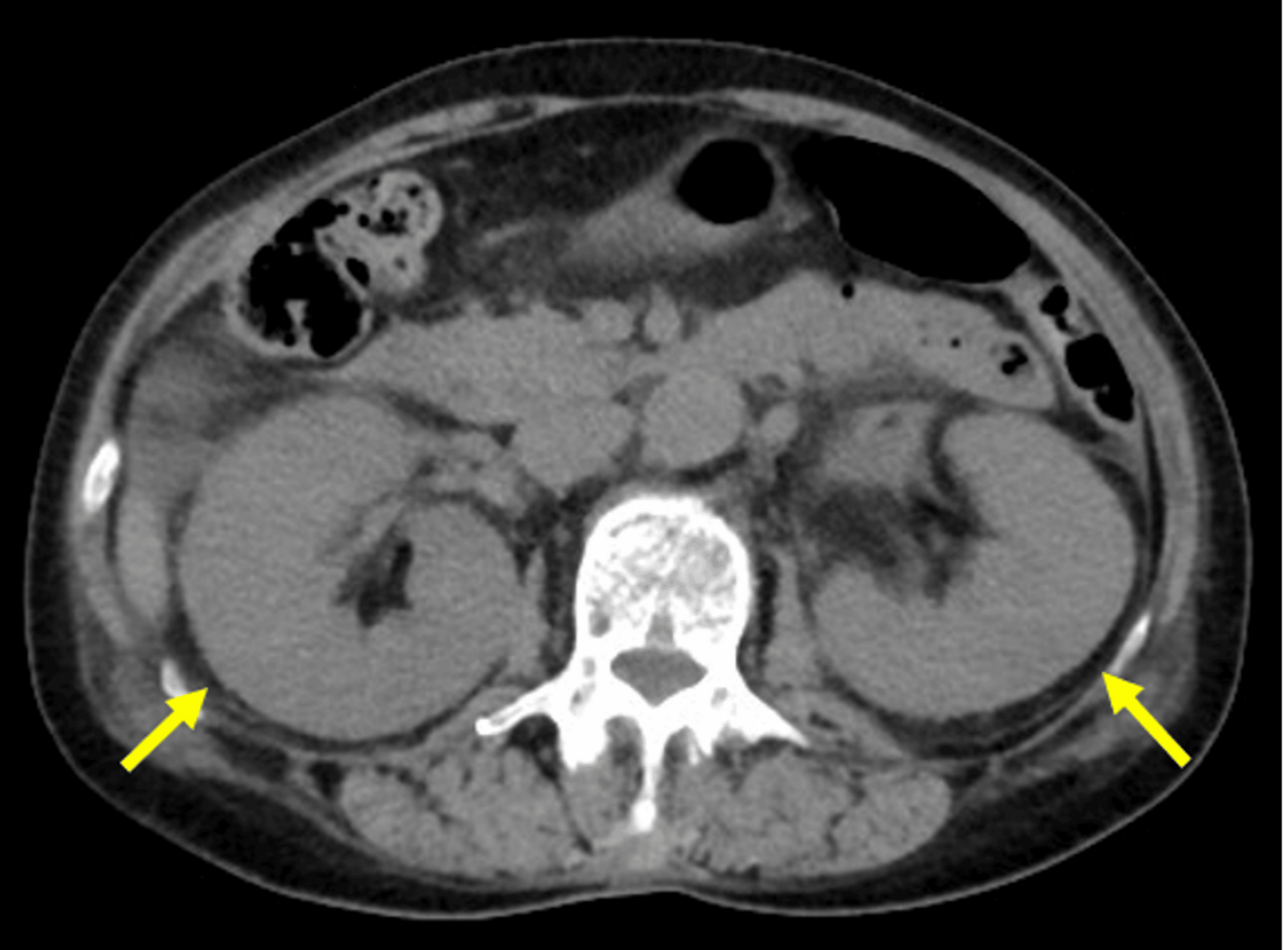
CT showing bilateral nephromegaly
